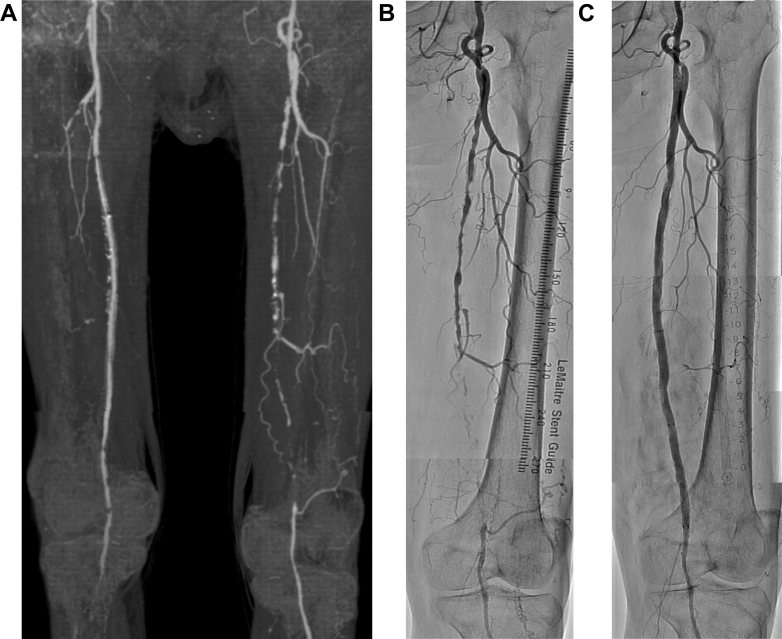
Initial EVT to the Left SFA TASC C lesion with short segment of CTO was treated with 2 SGs, inserted from the right femoral artery. (A) Preprocedural computed tomography findings. (B) Preprocedural and (C) postprocedural angiographic findings of left SFA. CTO = chronic total occlusion; EVT = endovascular therapy; SFA = superficial femoral artery; SG = stent graft; TASC = Transatlantic Inter-Society Consensus.
